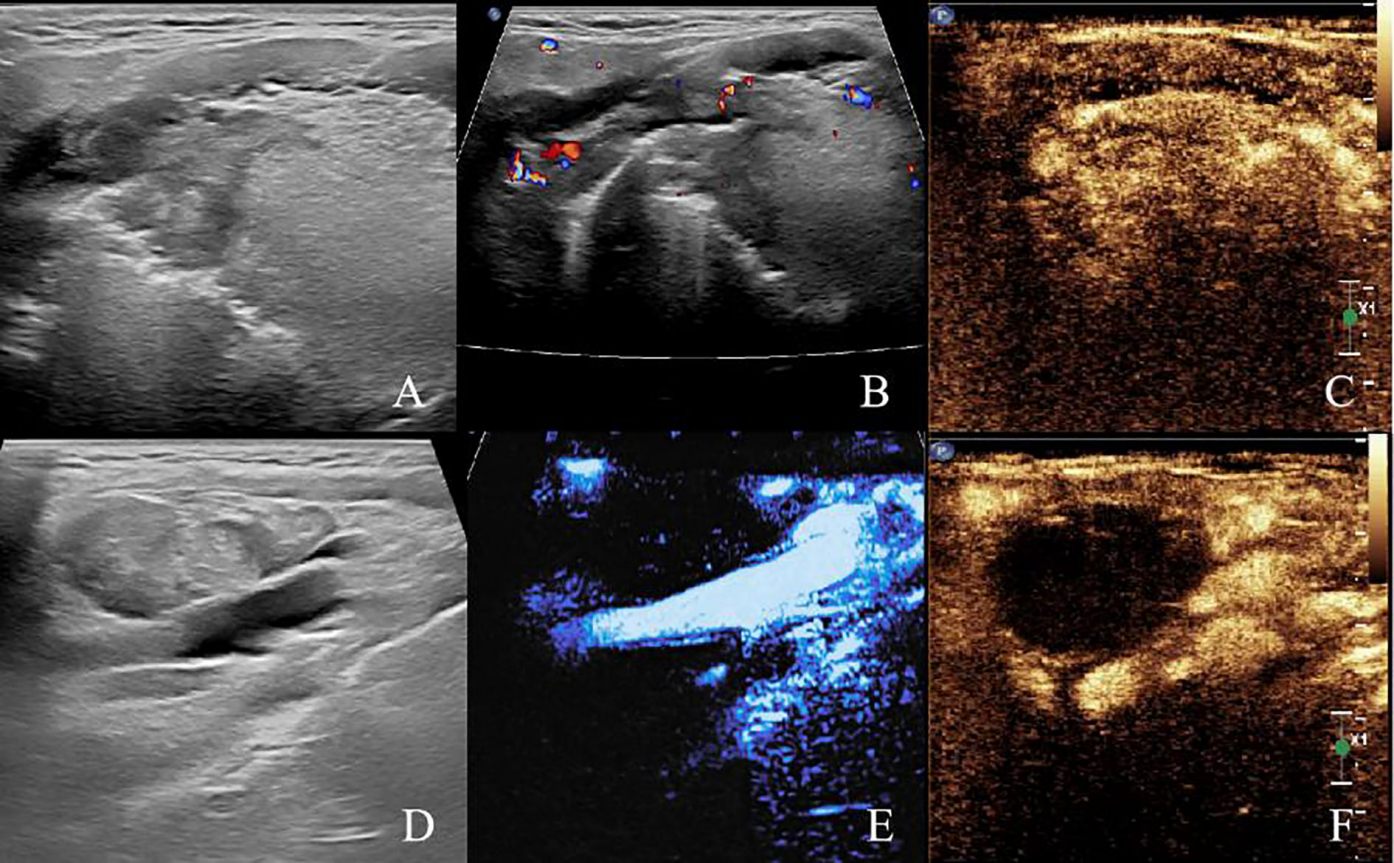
Pre-ablation submandibular ultrasonography (A) Two-dimensional ultrasound image of the right tongue base tumor. (B) CDFI image of the right tongue base tumor. (C) Preoperative CEUS image of the right tongue base tumor. (D) Two-dimensional ultrasound image of right cervical lymph node (level II). (E) CDFI image of right cervical lymph node (level II). (F) Preoperative CEUS image of right cervical lymph node (level II).
